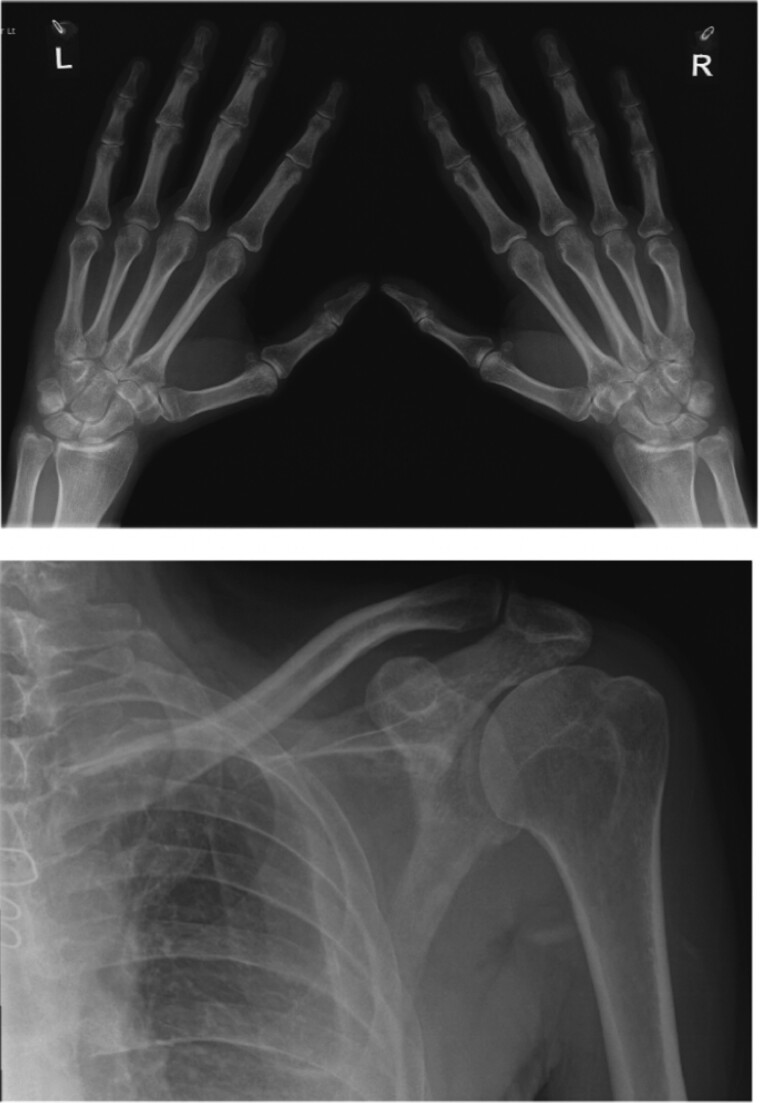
Radiographs of hands and left shoulder showing no evidence of erosive damage.
